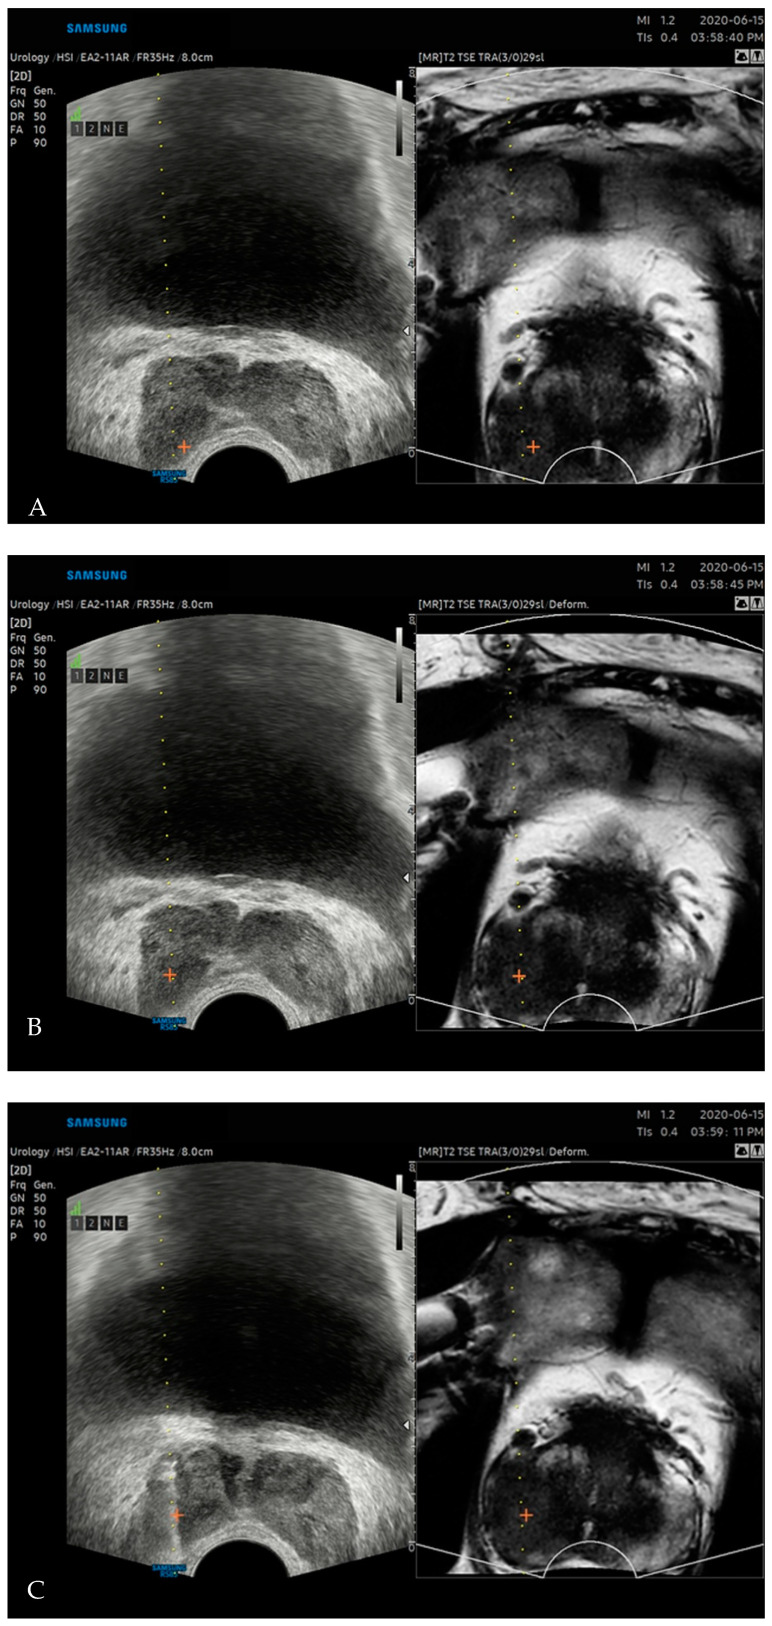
Fusion prostate biopsy of a 64-year-old male. Rigid registration between US and MRI (A) was performed, and index lesion was marked as cross on MRI, and transferred to the US. After nonrigid registration, deformed MRI and repositioning of index lesion markers in US were noted (B). Adenocarcinoma with a Gleason score of 8 (4 + 4) was confirmed by targeted biopsy performed on the index lesion (C). Trans-registration error decreased from 4.78 to 0.46 mm by switching from rigid to nonrigid registration.
